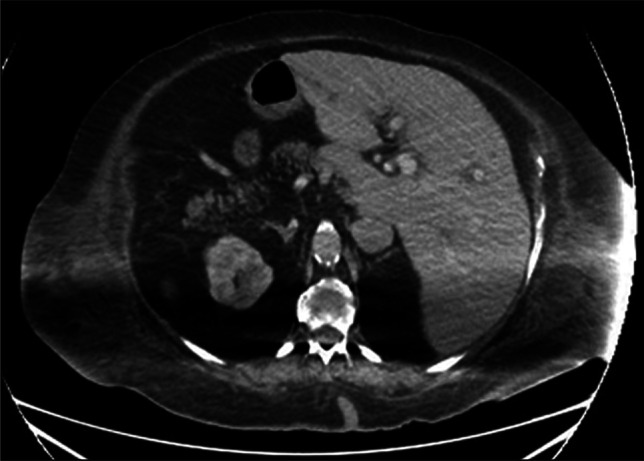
Axial computed tomography scan post endoscopic retrograde cholangiopancreatography demonstrates resolution of the dilated biliary system.
